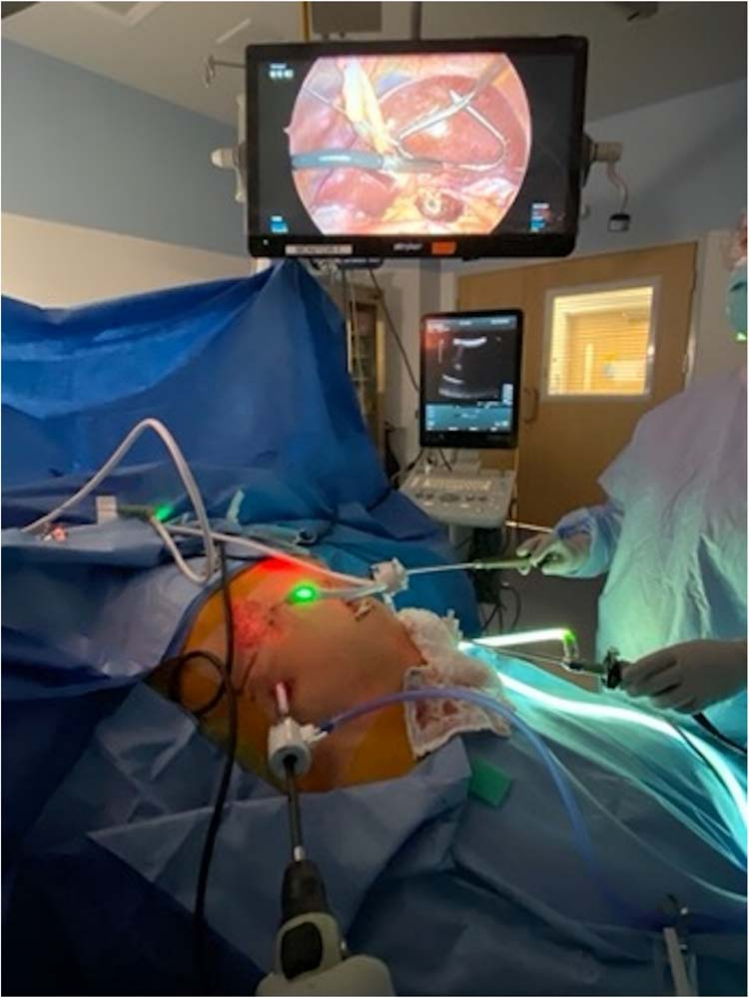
Patient positioned in reverse Trendelenburg to facilitate exposure of upper abdomen. Port placement: 12 mm port in right upper quadrant to facilitate laparoscopic ultrasound of liver, other 5 mm ports placed as needed for mobilization of liver. Laparoscopic image above patient demonstrates left lobe of liver elevated with retractor, laparoscopic ultrasound probe evaluating left lobe of the liver, and burned area visible in caudate lobe below.
